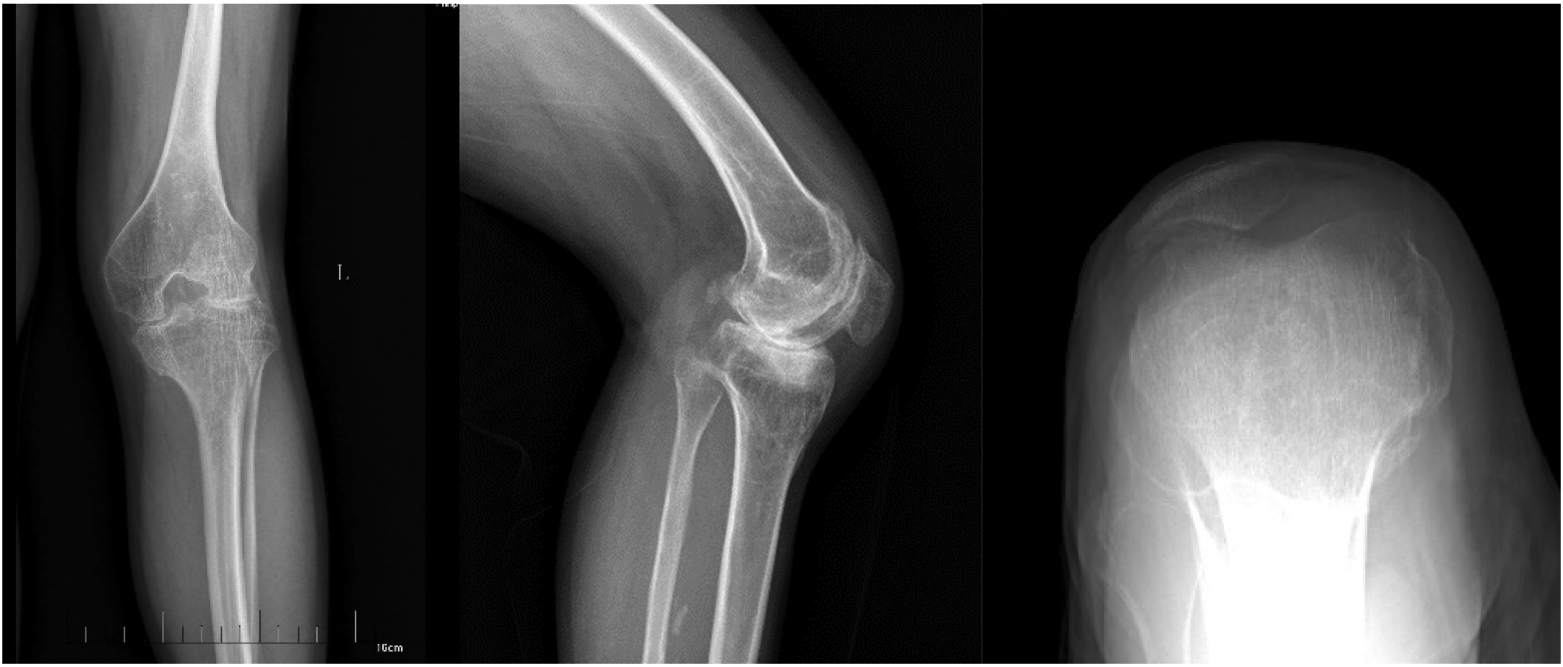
Radiography of a patient with hemophilic knee arthritis.
